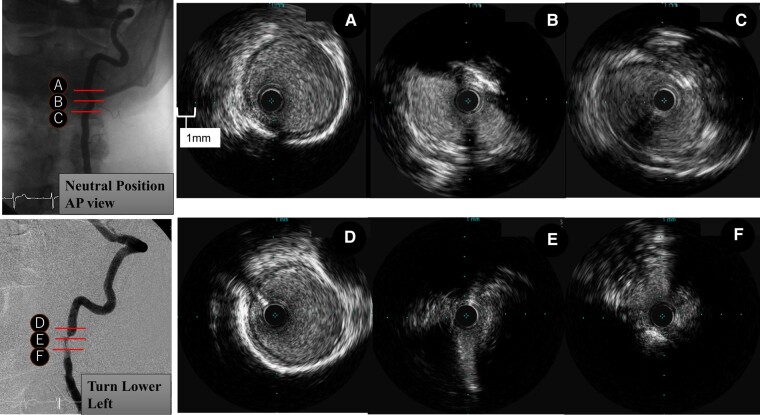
Intravascular ultrasound (IVUS) of the vertebral artery. (*A* and *B*) IVUS of the left vertebral artery in the normal cephalic position [*A*: normal site, *B*: the most stenotic site (calcified lesion at ∼90°), *C*: a partially attached plaque]. (*D–F*) IVUS with left inferior rotation (*D*: normal site, *E*: a highly stenotic lesion with the calcified lesion, (*F*): plaque adhesion with worsened stenosis).
